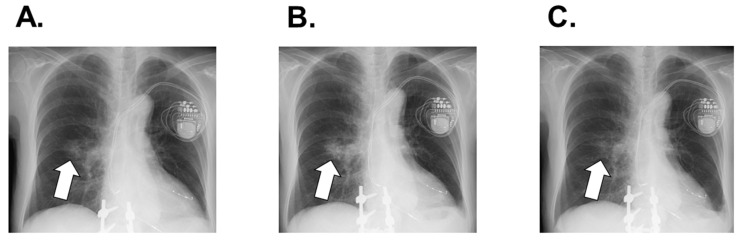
Post-discharge chest X-ray ((A), at discharge implying recurrent right lung consolidation; (B), 1 month later showing worsening consolidation; (C), following 2 months of osimertinib therapy proving remission of consolidation). White arrows show recurrent consolidation.
